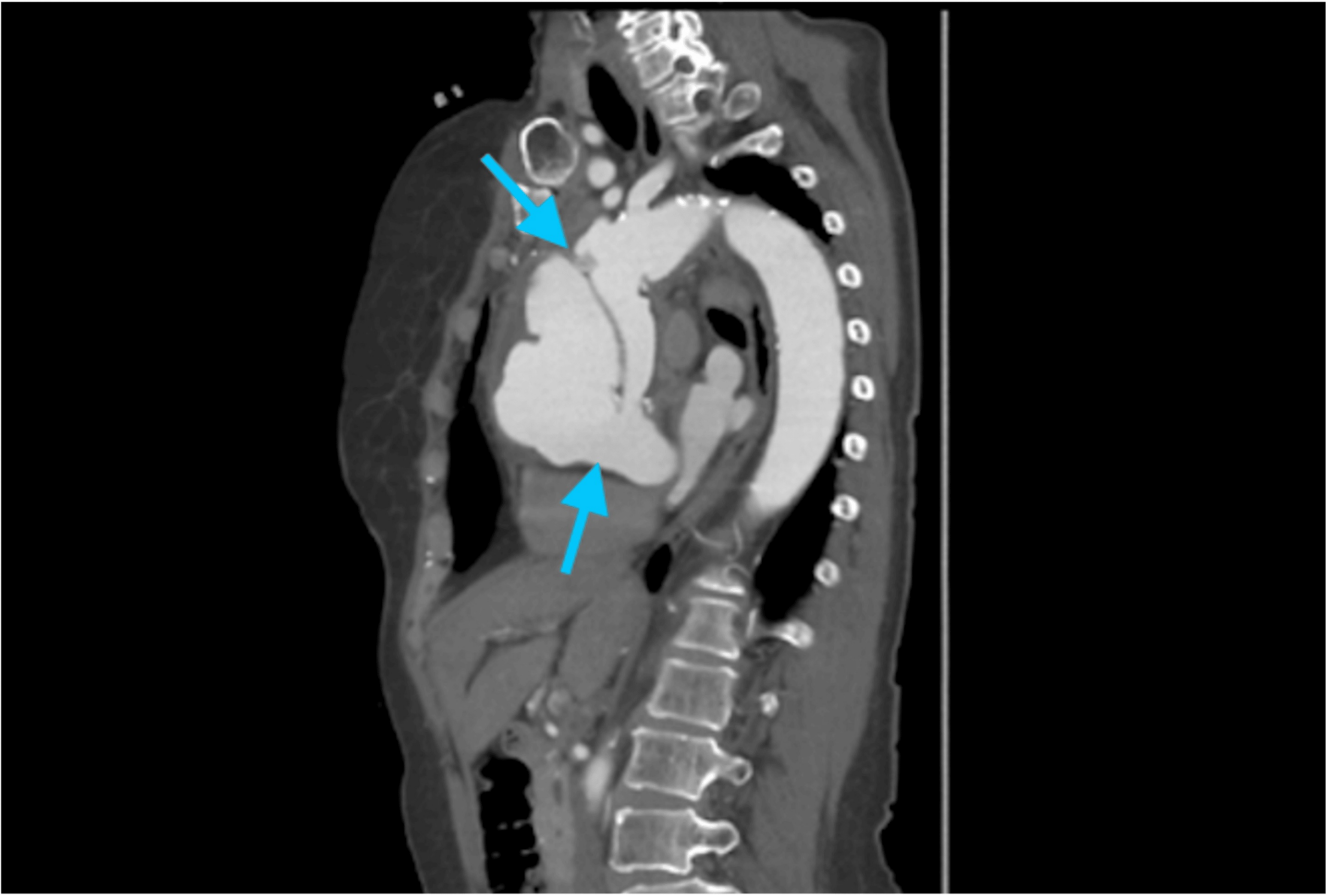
Sagittal CT image Proximal entry (lower blue arrow) and distal re-entry (upper blue arrow) into a false aneurysm of the ascending aorta following a previously placed ascending aortic graft.
